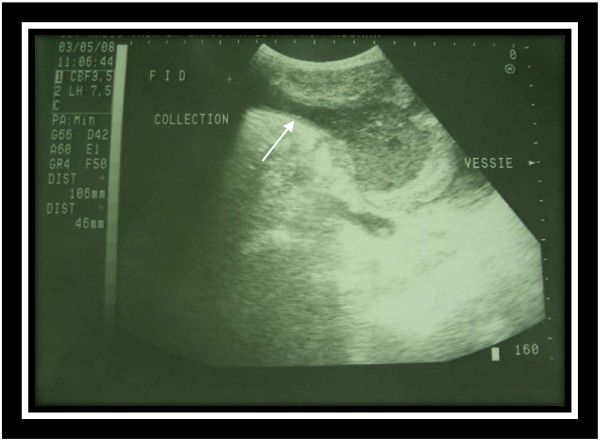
Longitudinal ultrasonography of the lower abdomen shows a heterogeneous urachal collection, extending from the periumbilical region to the dome of the bladder.
